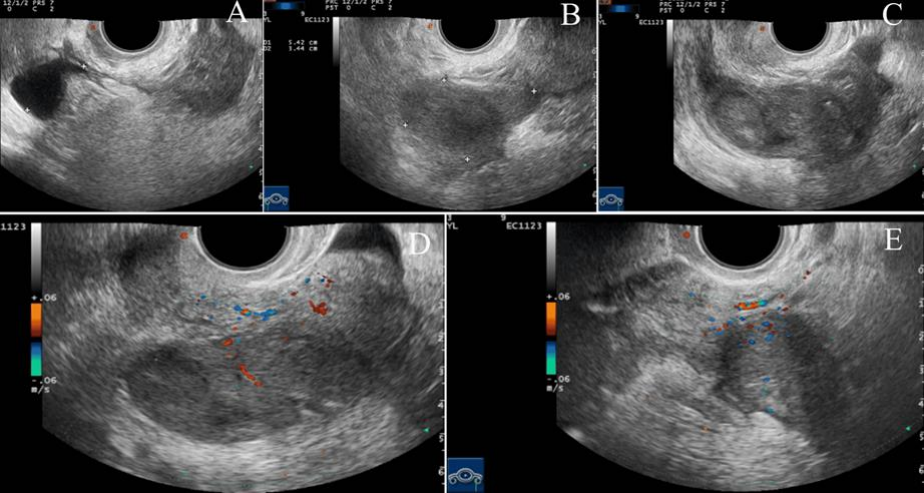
transvaginal ultrasound: A) sagittal: 29mm free fluid (*); B) right: 54×34mm hypoechoic mass, angular margins, flow; C) left: 74×34mm heterogeneous lesion, 3-5mm foci; D) left: moderate flow, RI 0.42; E) right: marked peri-/central flow
